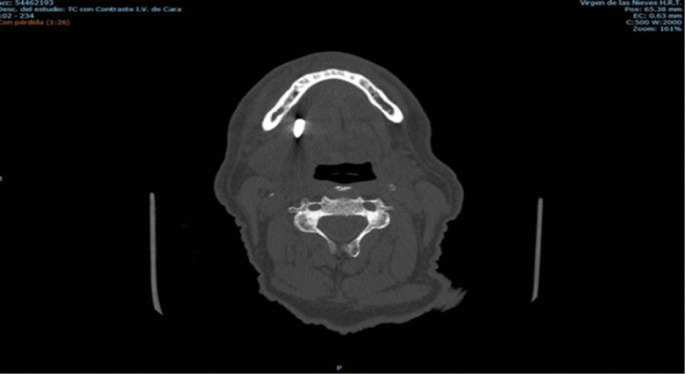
CT images of implant displacement.
